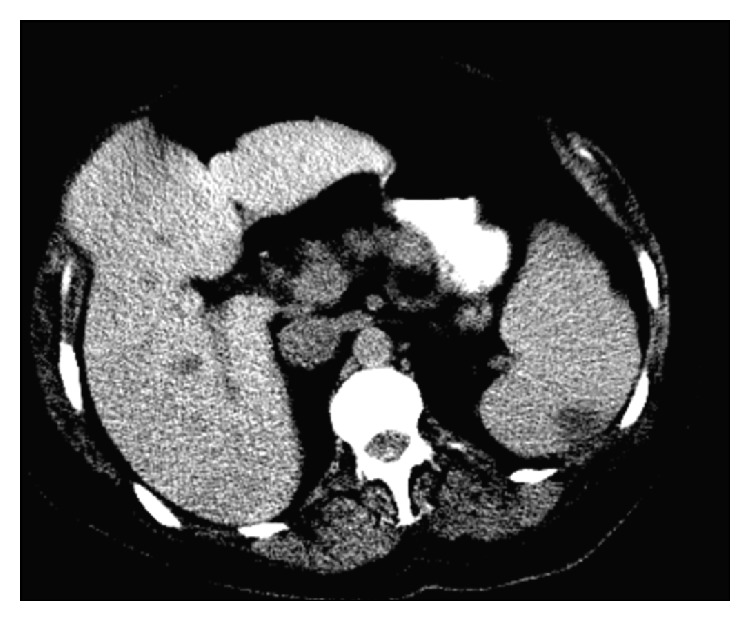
CT scan of the abdomen, depicting splenic infarct.
